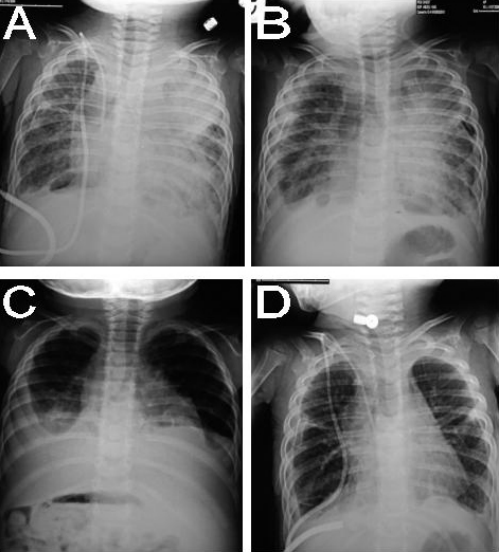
Pulmonary Lymphangiectasia. Chest radiographs, AP views. Radiological findings occurring during the clinical course of PL. A and B: over time progression of hazy perihilar infiltrates on the left lung. C: important bilateral pleural effusion. D: after pleurodesis, bilateral lung hyperinflation with interstitial and septa thickening are evident, and a mild degree of pleural effusion remains.
